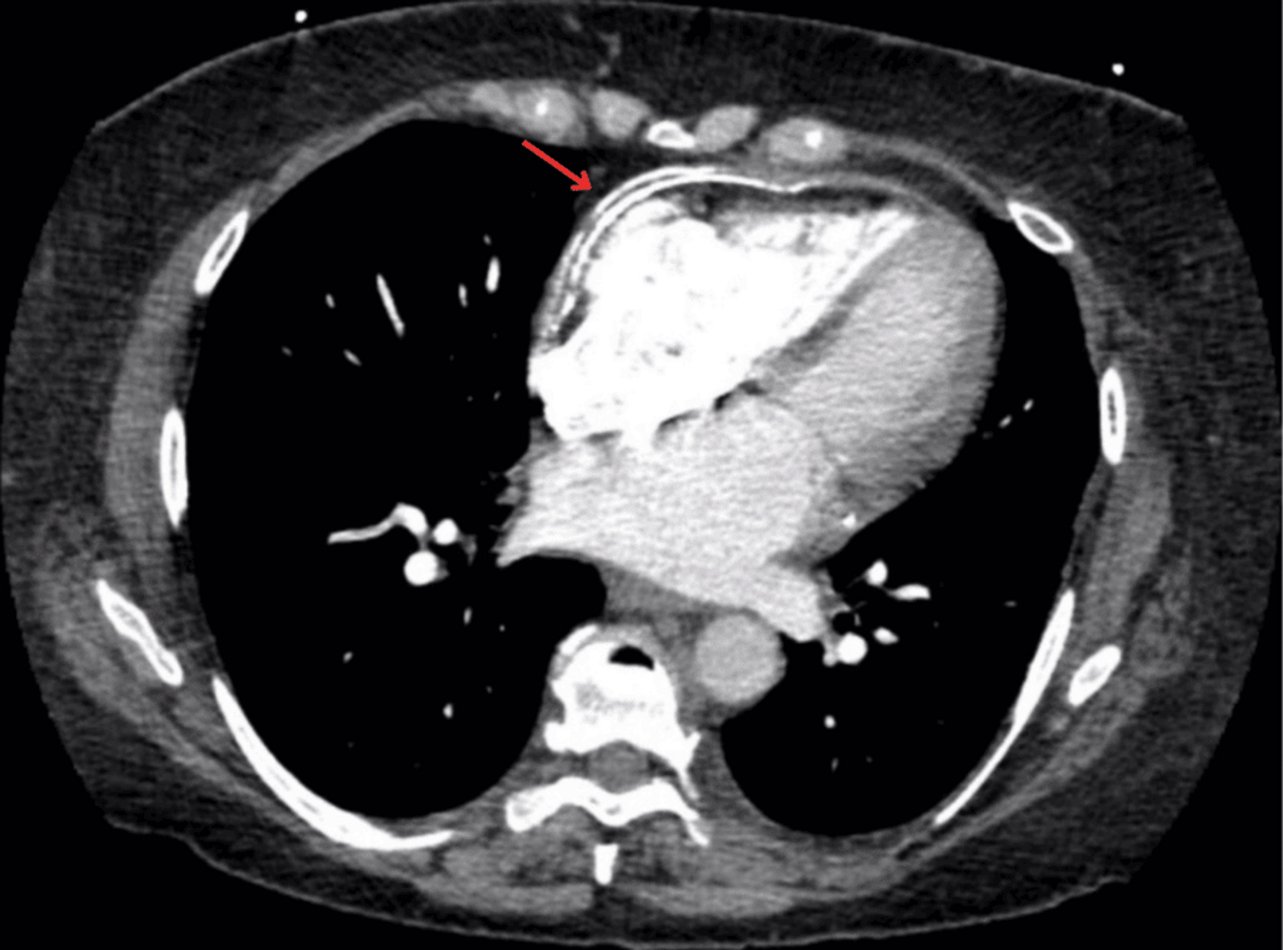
Computed tomography of the chest  showing extensive pericardial calcification, most severe along the right ventricular lateral wall, with small pericardial thickening/effusion with a maximum thickness of 7 mm.
